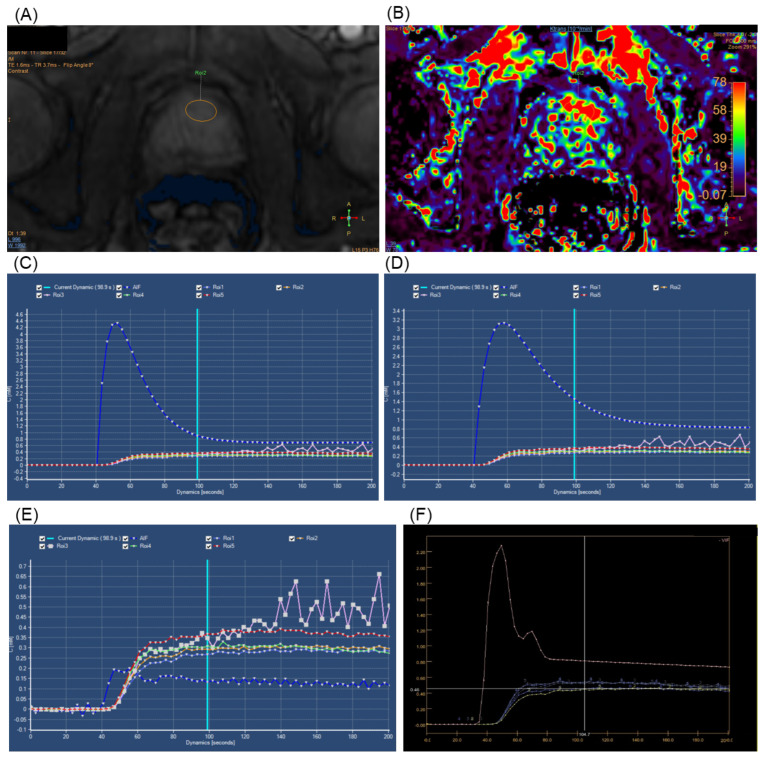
Comparison of arterial input function (AIF) time-concentration curves between solutions (A to E, ISP; F, GenIQ) and presets in a 66-year-old man with prostate cancer in the left middle transition zone (Gleason score 6). Multiple ROIs from tumor are presented in various colors. (A) Axial DCE-MRI image on IntelliSpace Portal (ISP) showing placement of a region of interest (ROI) within the index tumor. (B) Corresponding K^trans^ map with the tumor ROI overlaid. (C) The ISP medium preset AIF, showing a peak arterial concentration of approximately 4.4 mM. The calculated K^trans^ (volume transfer constant) was 0.1/min. (D) ISP short preset AIF, showing a lower arterial peak of approximately 3.2 mM; The calculated K^trans^ was 0.1/min. (E) Individual AIF in ISP; AIF graph is in blue and tumor concentration graphs from 5 tumor ROIs are in other colors. The average calculated K^trans^ value was 2.8/min (F) GenIQ population-based AIF, displayed with absolute concentration on the *y*-axis, with an arterial peak of approximately 2.3 mM. The calculated K^trans^ was 0.48/min. Differences in AIF preset and type are assumed to contribute to variability in estimated perfusion parameters.
